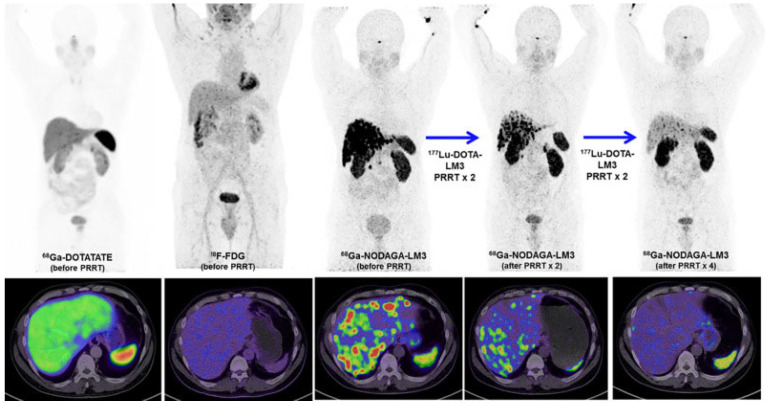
A patient with well-differentiated, non-functioning metastatic pancreatic NEN. [^68^Ga]Ga-DOTA-TATE PET/CT showed lesions in liver and lymph nodes with extremely low uptake (leftmost image), not exhibiting significant glucose hypermetabolism (second image from left). [^68^Ga]Ga-NODAGA-LM3 PET/CT instead showed disseminated metastases, with intense uptake in liver and lymph nodes (third image from left). After four cycles of [^177^Lu]Lu-DOTA-LM3 PRRT, restaging [^68^Ga]Ga-NODAGA-LM3 PET/CT showed excellent response (partial remission, rightmost image).This research was originally published in *JNM* 2020 ([64]; https://jnm.snmjournals.org/content/jnumed/62/11/1571/F6.large.jpg).
